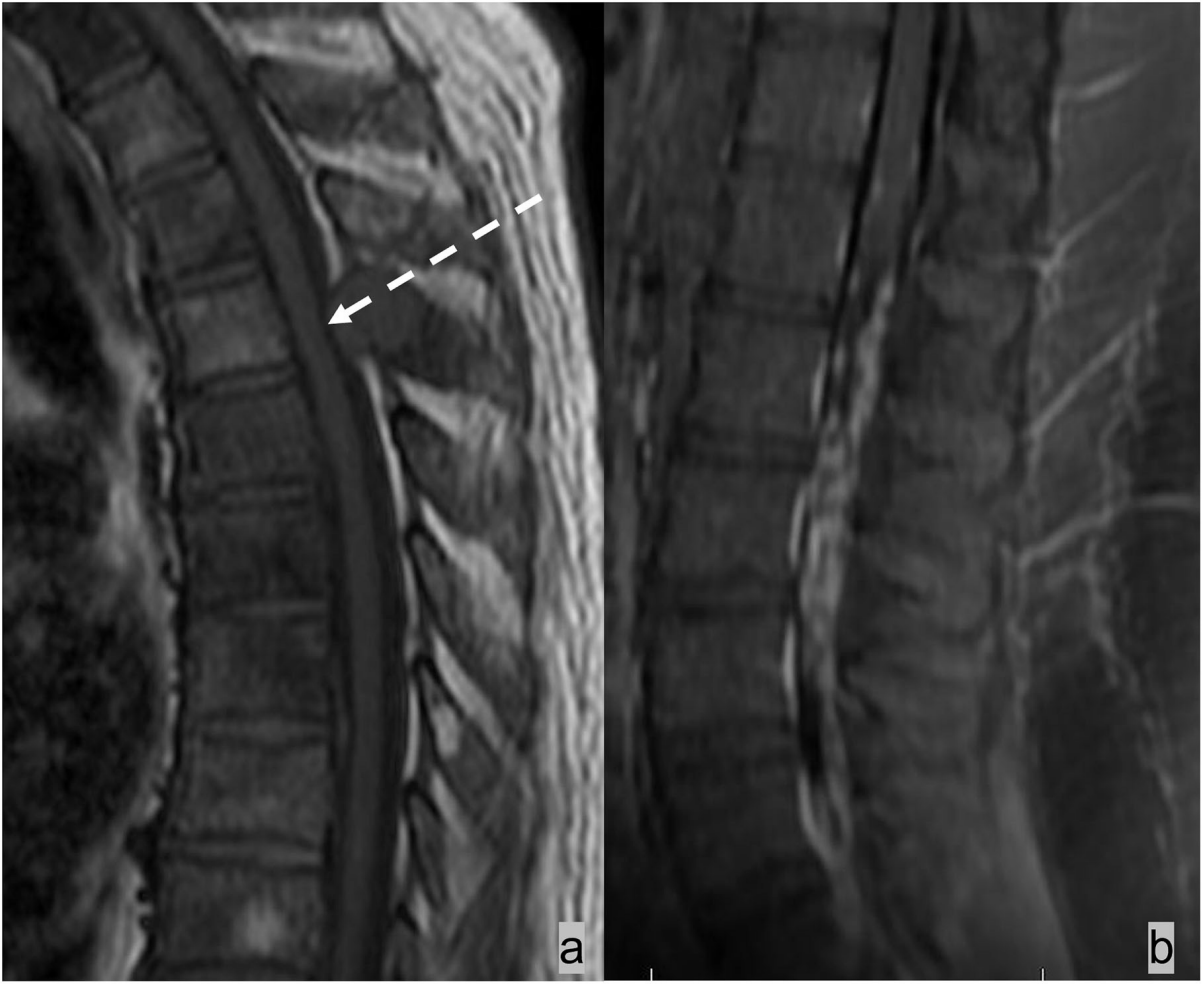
Two different patients with (a) epidural and (b) intradural leptomeningeal metastases. (a) Patient 1 had lung cancer with spinal metastases. Sagittal T1w sequence demonstrated multiple marrow-replacing bony metastases. The metastatic lesion at the T4 spinous process (white arrow) effaces the epidural fat and results in inward displacement of the thecal sac. (b) Patient 2 had neoplastic meningitis from metastatic lymphoma. MRI of the lumbar spine demonstrated extensive nodular leptomeningeal enhancement on the post-contrast T1w image.
